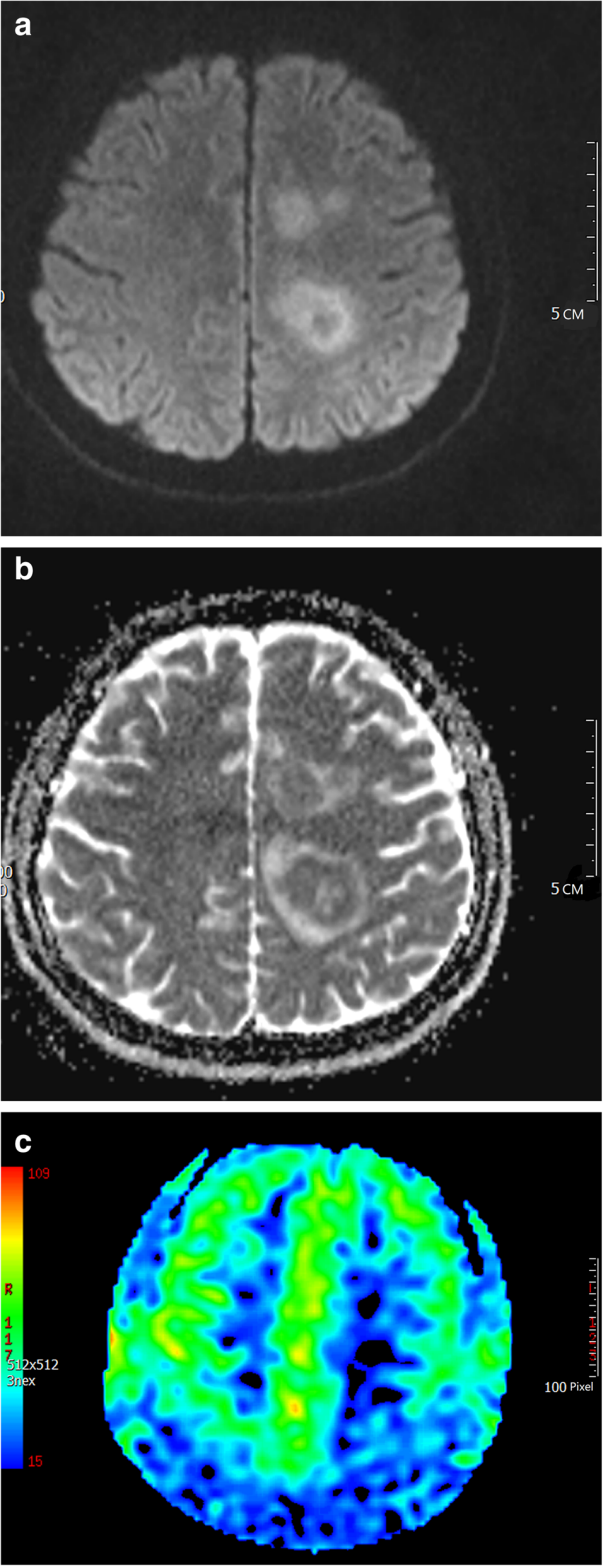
Multimodal MR (DWI/ADC and  3D-pCASL CBF) images of an AR-PCNSL patient. A young man. a. Irregular lesions were found periventricular of the left lateral ventricle, with high signal on DWI, low signal on ADC b. c The lesion showed decreased CBF on 3D-pCASL. We used GE MR ADW4.6 workstation perfusion mode for correction and noise reduction to obtain CBF perfusion images
